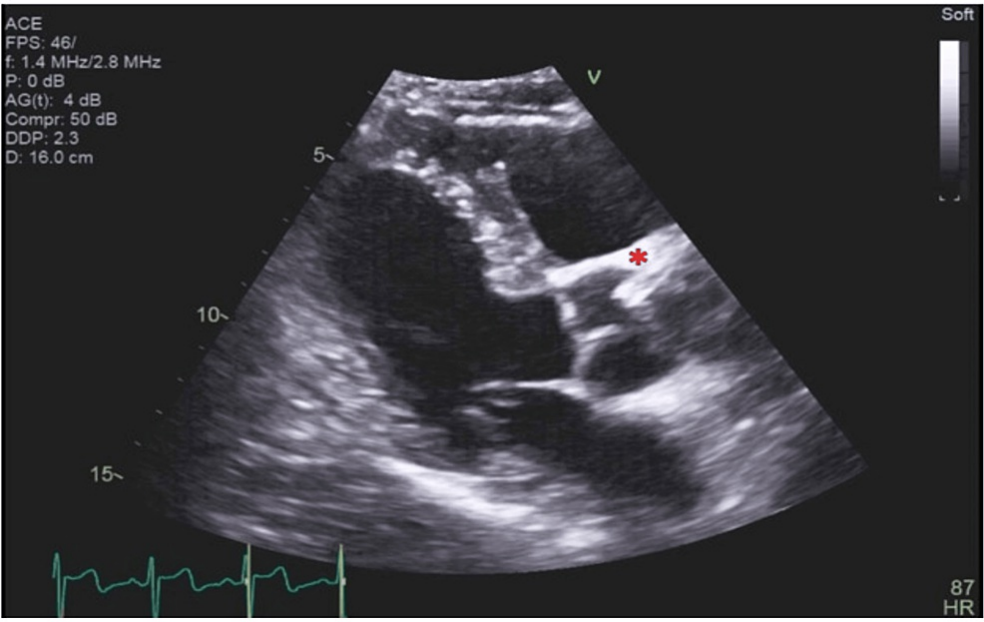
Transthoracic echocardiogram in a parasternal long-axis view Transthoracic echocardiogram (TTE) in a parasternal long-axis (PLAX) view. A long, tubular, echodense structure can be seen arising from the right coronary sinus and into the aortic root (marked with red asterisk).
